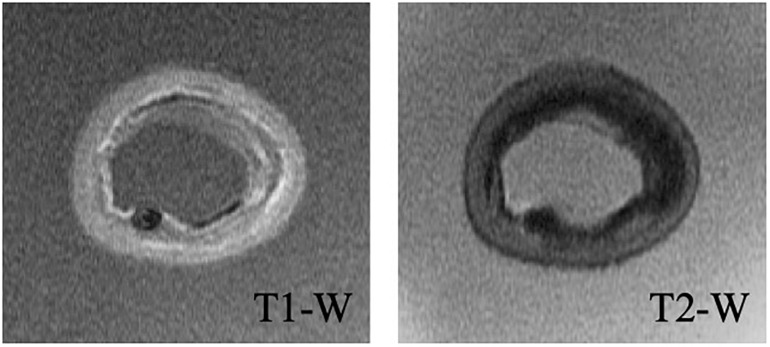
Representative T1- and T2-weighted MRI images of cross-section #9.
